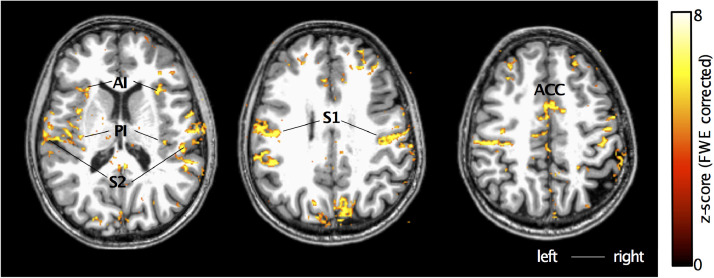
Cortical activation patterns (z-score>3.08, FWE corrected) in response to thermonoxious stimulation of the thenar eminence *of* Subject 2 overlaid onto structural T1-weighted space.
